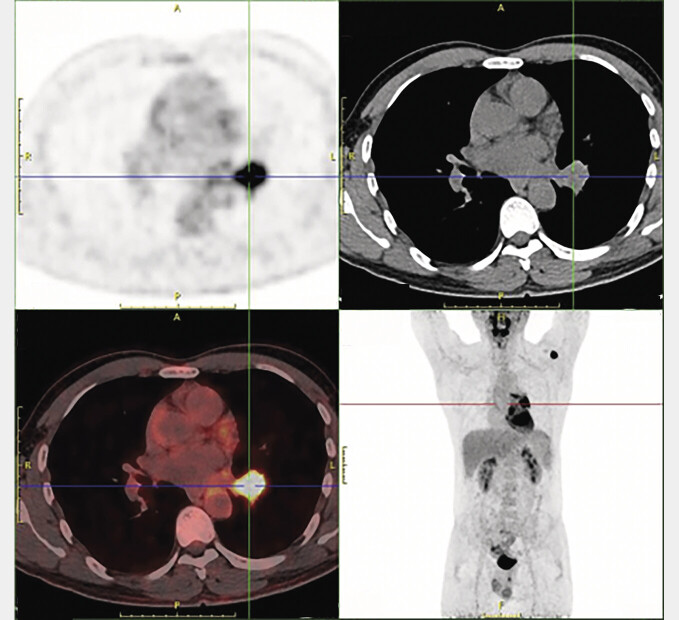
PET-CT showed hypermetabolic lesions in the lower lobe of the left lung adjacent to the hilum.
